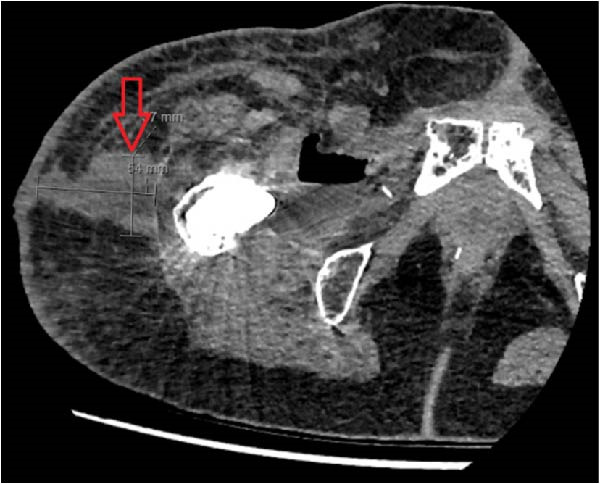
CT right hip, red arrow indicating fluid around the hip joint.
